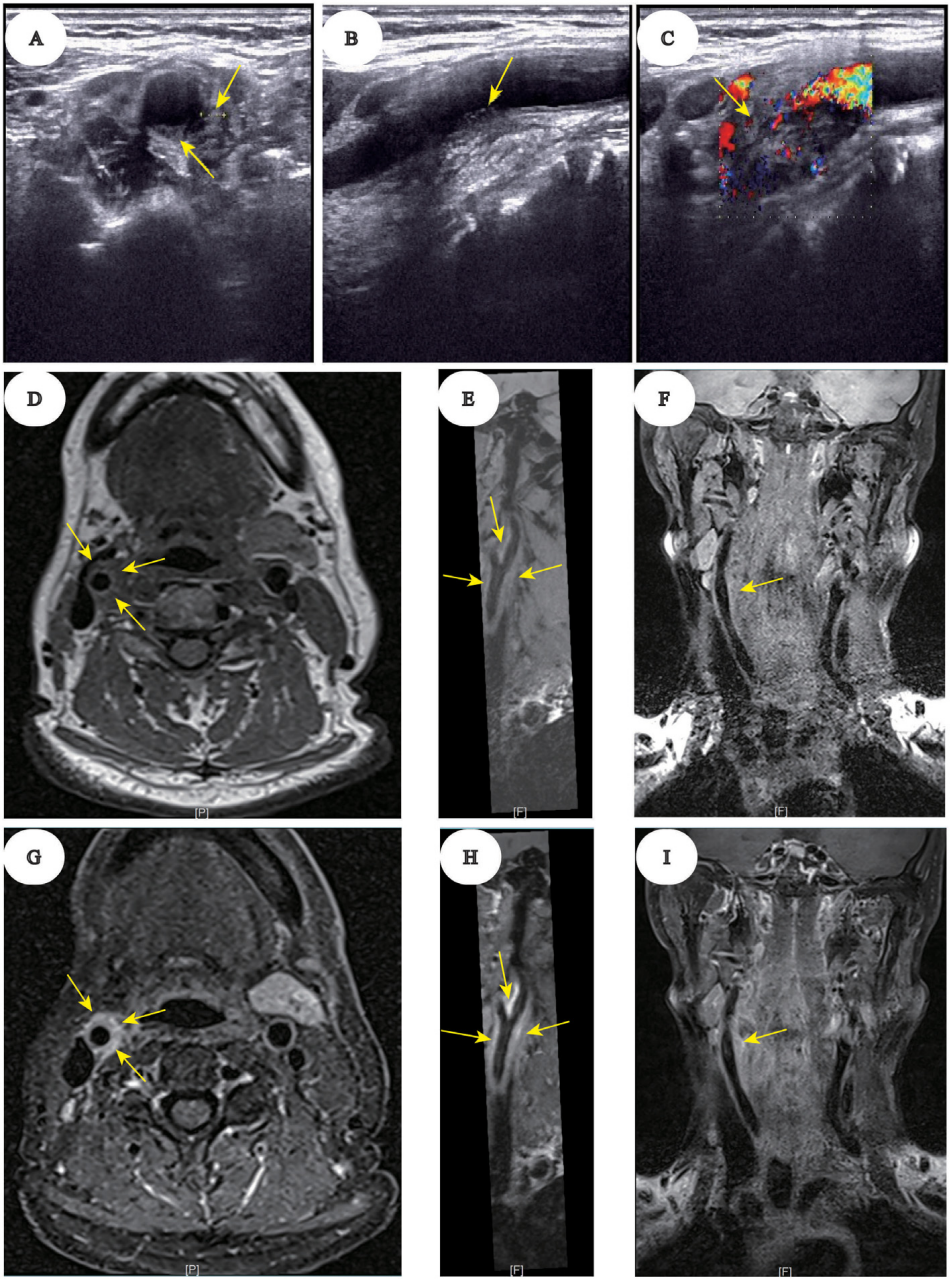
Ultrasound and MRI imaging of right carotid artery wall thickening in TIPIC syndrome. Ultrasound imaging in axial (A), sagittal (B), and coronal (C) planes. Eccentric thickening of the arterial wall is observed from the upper right CCA to the bifurcation, particularly on the posteromedial wall. The boundary between the arterial wall and surrounding tissue is indistinct. Pre-contrast MRI vessel wall imaging in axial (D), sagittal (E), and coronal (F) planes. Mild luminal narrowing and uniform wall thickening are seen in the mid-to-distal segments of the right CCA, extending into the ICA. Post-contrast MRI vessel wall imaging in axial (G), sagittal (H), and coronal (I) planes. Significant homogeneous enhancement of the thickened vessel walls is evident.
